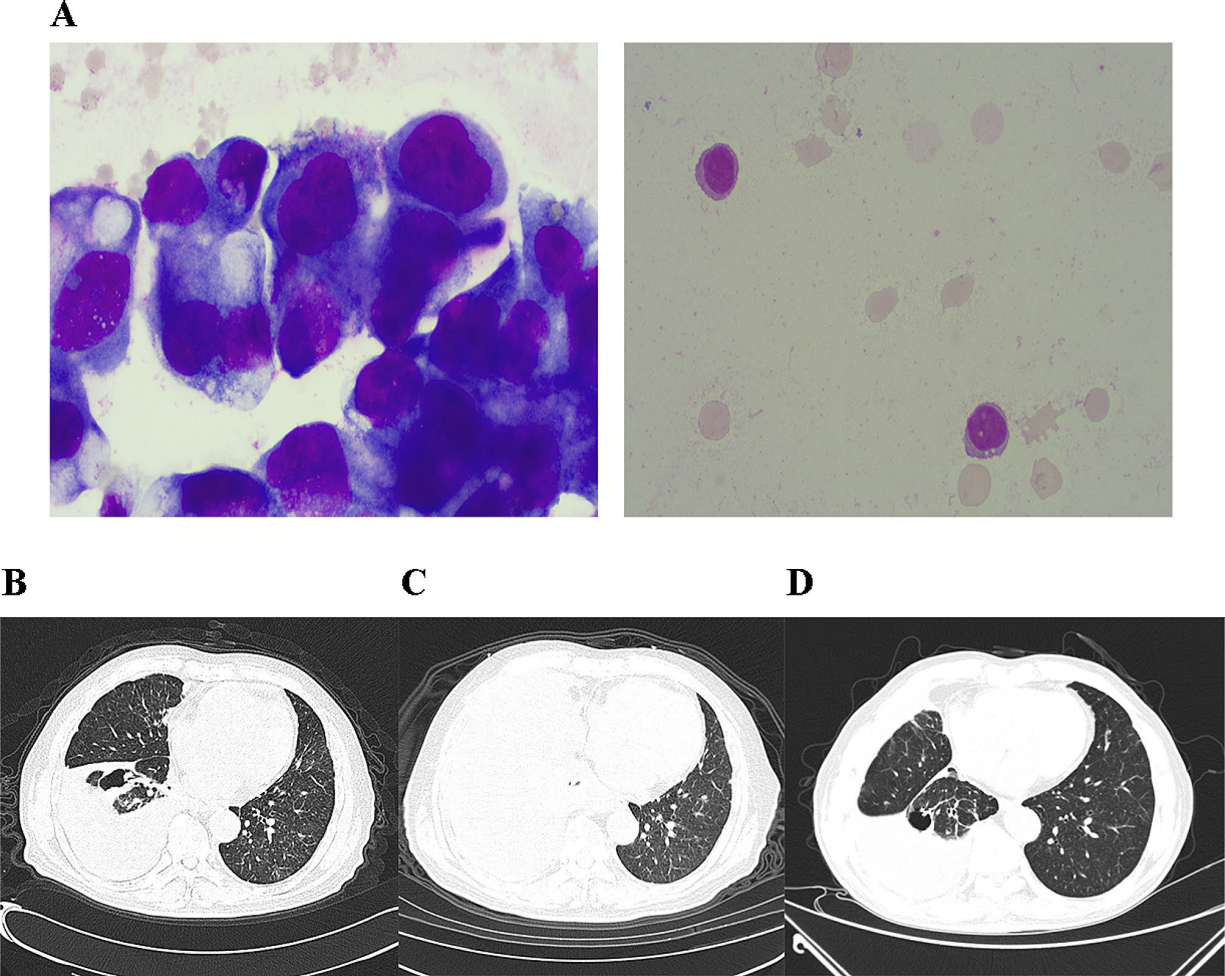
Hydrothorax Cytology examination of and chest CT scan of the patient. (A) Cytology examination of hydrothorax; (B) Chest CT scan at the diagnosis time of LUAD; (C) Chest CT scan at the beginning of EGFR-TKI treatment; (D) Chest CT scan after 8 months of EGFR-TKI treatment
